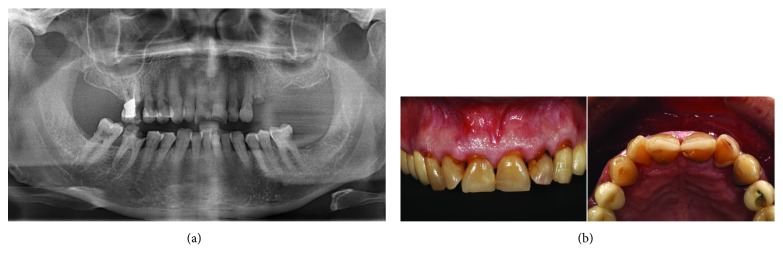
(a) Initial panoramic radiograph. (b) Clinical view of the anterior teeth. The roots are subgingivally and peripherally decayed.
